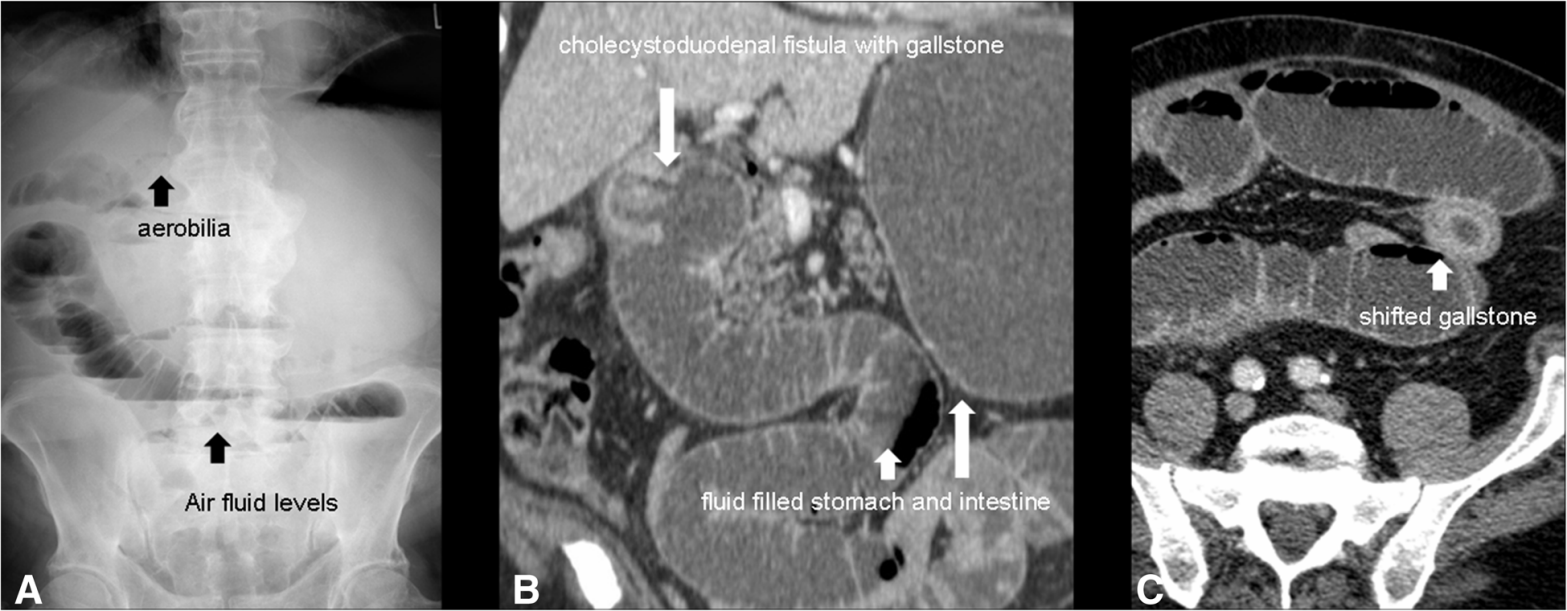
Case 2, A: X-ray with multiple air fluid levels as a sign of ileus, B: double-oblique MPR of the CT scan showing the gallstone within a cholecystoduodenal fistula and a fluid filled stomach and proximal intestine C: axial slice of the CT scan with delineation of the shifted gallstone in the jejunum.
